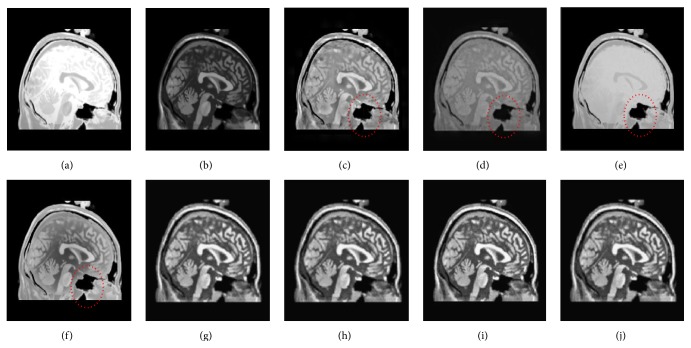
The fusion results of different fusion methods for the second set of CT and MRI images: (a), (b) Source images; fused images by (c) DWT, (d) GP, (e) PCA, (f) GF, (g) CT, (h) NSCT-1, (i) NSCT-2, and (j) the proposed method.
